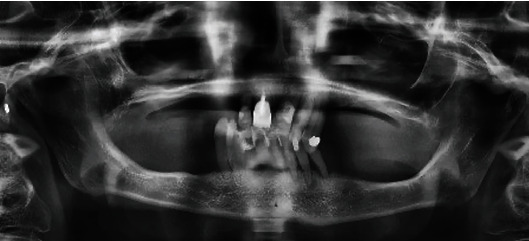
Panoramic radiograph (OPG) image depicting the patient's residual dentition not worthy of preservation and a severe generalized horizontal bone loss of 80-90%.
